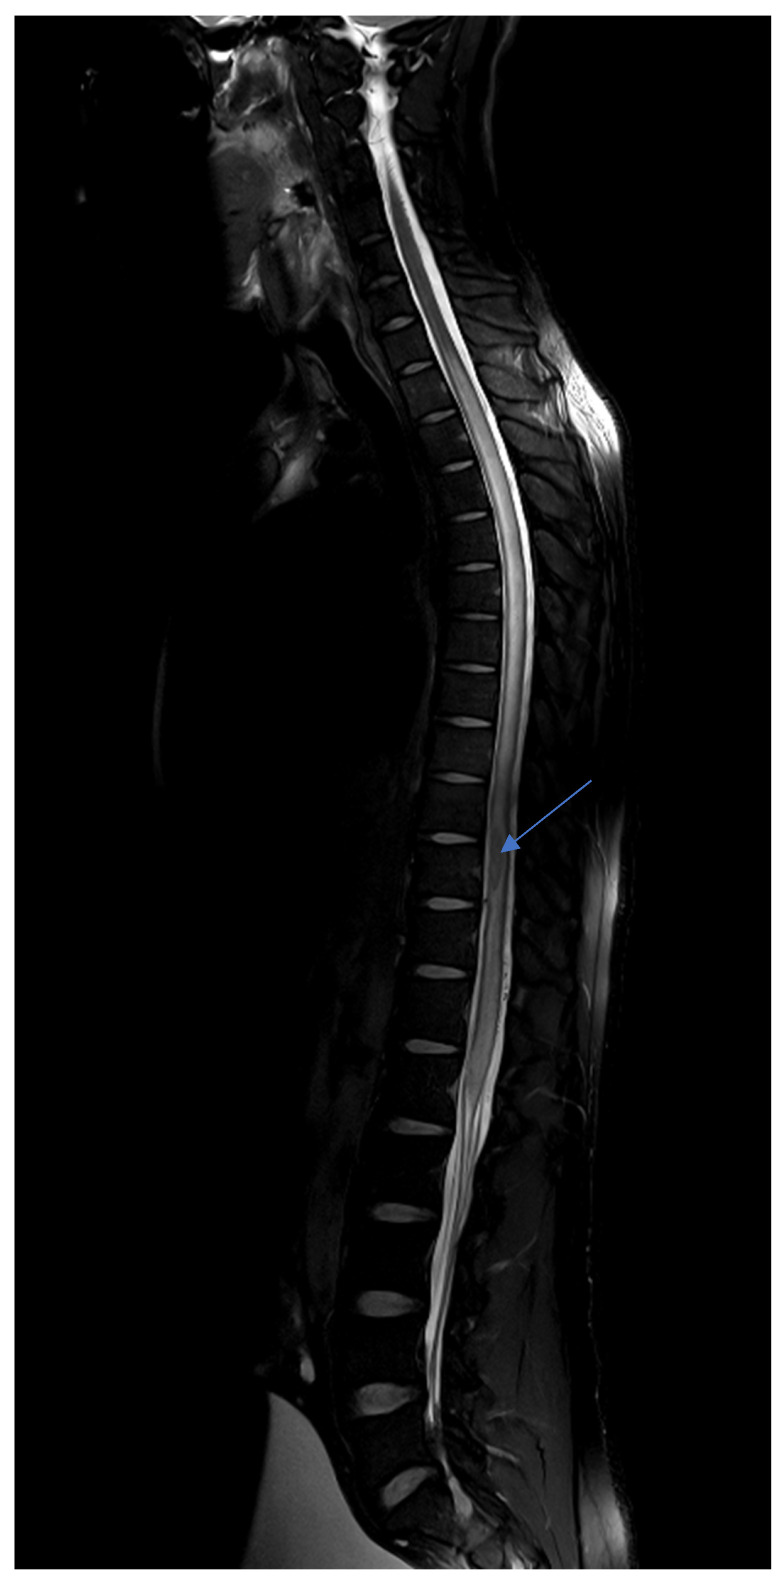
T2-weighted MRI shows an anterior intramedullary abscess at the level of the inferior border of the D9 vertebral body and the D10 vertebral body. A central intramedullary T2 hyperintense signal abnormality is observed, extending from the inferior aspect of C7 to the conus medullaris, associated with a mild to moderate diffuse enlargement of the spinal cord over the affected segment.
